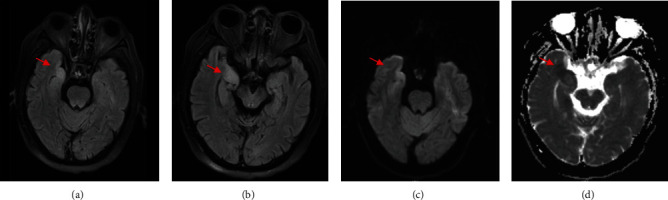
MRI findings in a patient with herpes simplex virus encephalitis. (a) Flair sequence shows high signal in the right temporal lobe. (b) Flair sequence showing high signal in the right hippocampus. (c) DWI sequence showing high signal in the right temporal lobe. (d) Right temporal lobe low signal. The red arrow indicates the lesion area.
